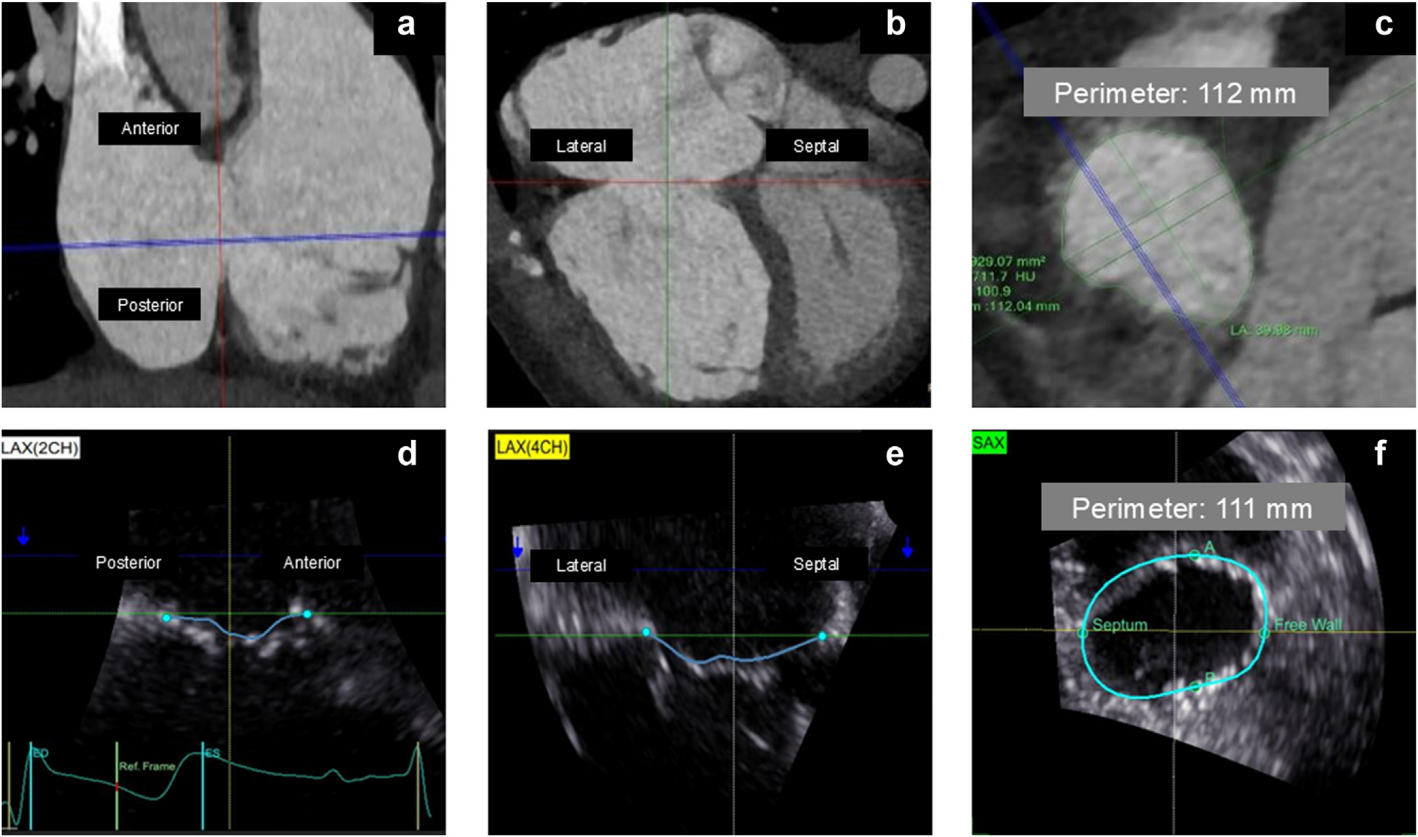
Representative CT vs. 3D TEE images. (a) Tricuspid annulus plane in the anterior and posterior view (2-chamber). (b) Tricuspid annulus plane in the lateral and septal view (4-chamber). (c) Tricuspid annulus plane in the en face view with perimeter measurement. (d) The 3D TEE view of the tricuspid annulus plane in the 2-chamber view. (e) The 3D TEE view of the tricuspid annulus plane in the 4-chamber view. (f) The 3D TEE view of the tricuspid annulus plane in the en face view with perimeter measurement Abbreviations: CT, computed tomography; ED, end diastole; ES, end systole; 3D, three-dimensional; LA, long axis; SA, short axis; TEE, transesophageal echocardiography.
